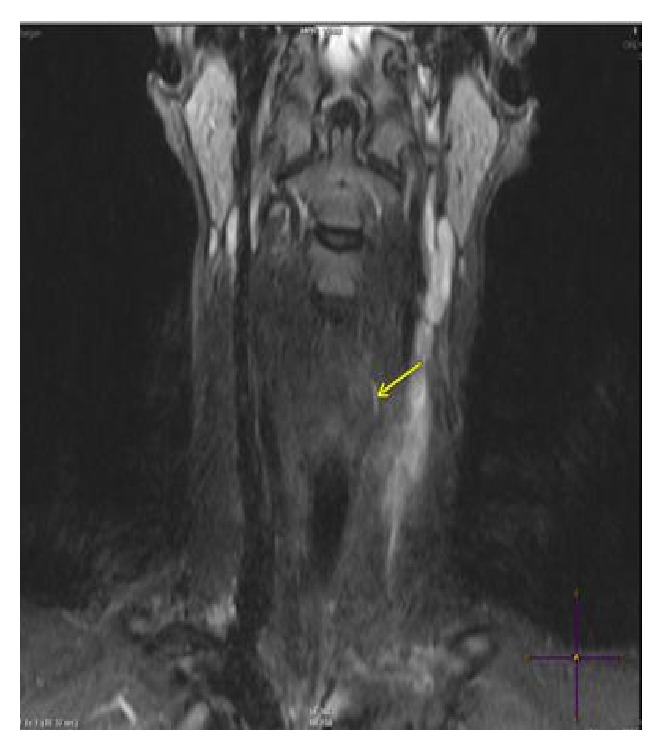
T1 MRI coronal view after injection.
